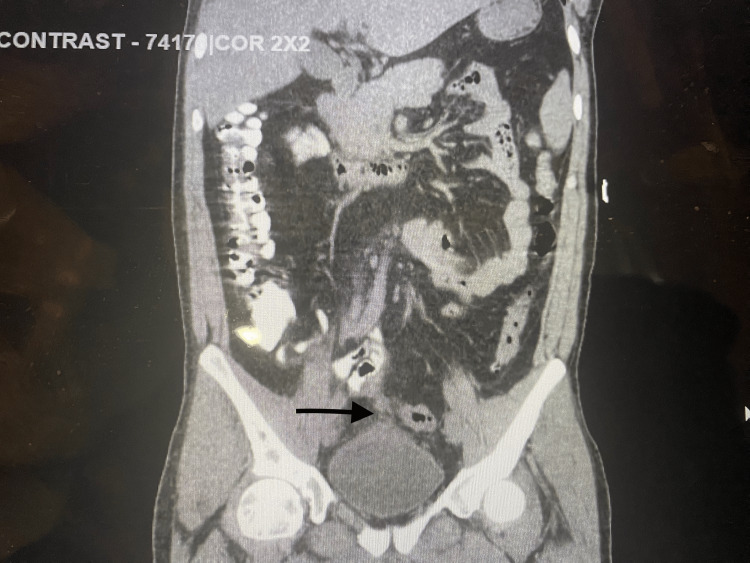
CT of the abdomen/pelvis with oral contrast. The black arrow indicates the colovesical fistula.
